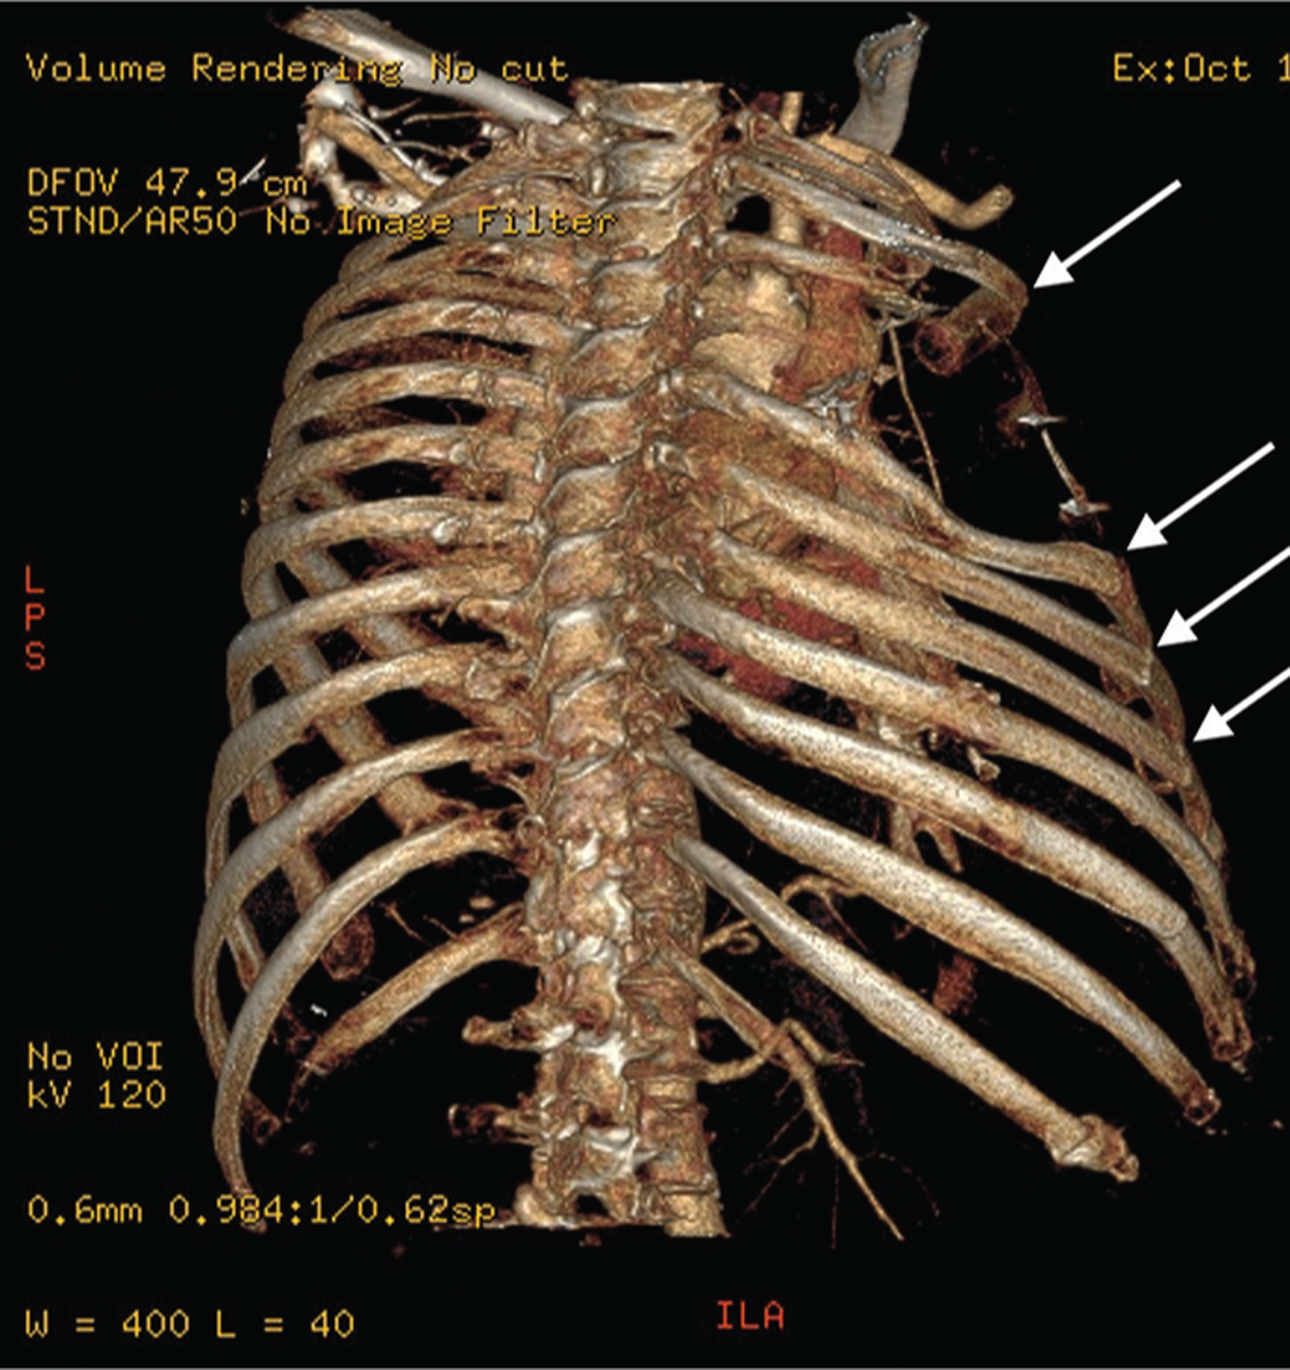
Three-dimensional volume rendering of computed tomography. Image displays the partial resections of ribs 3 and 4. Arrows indicate the lateral fractures of ribs 2, 5, 6, and 7
